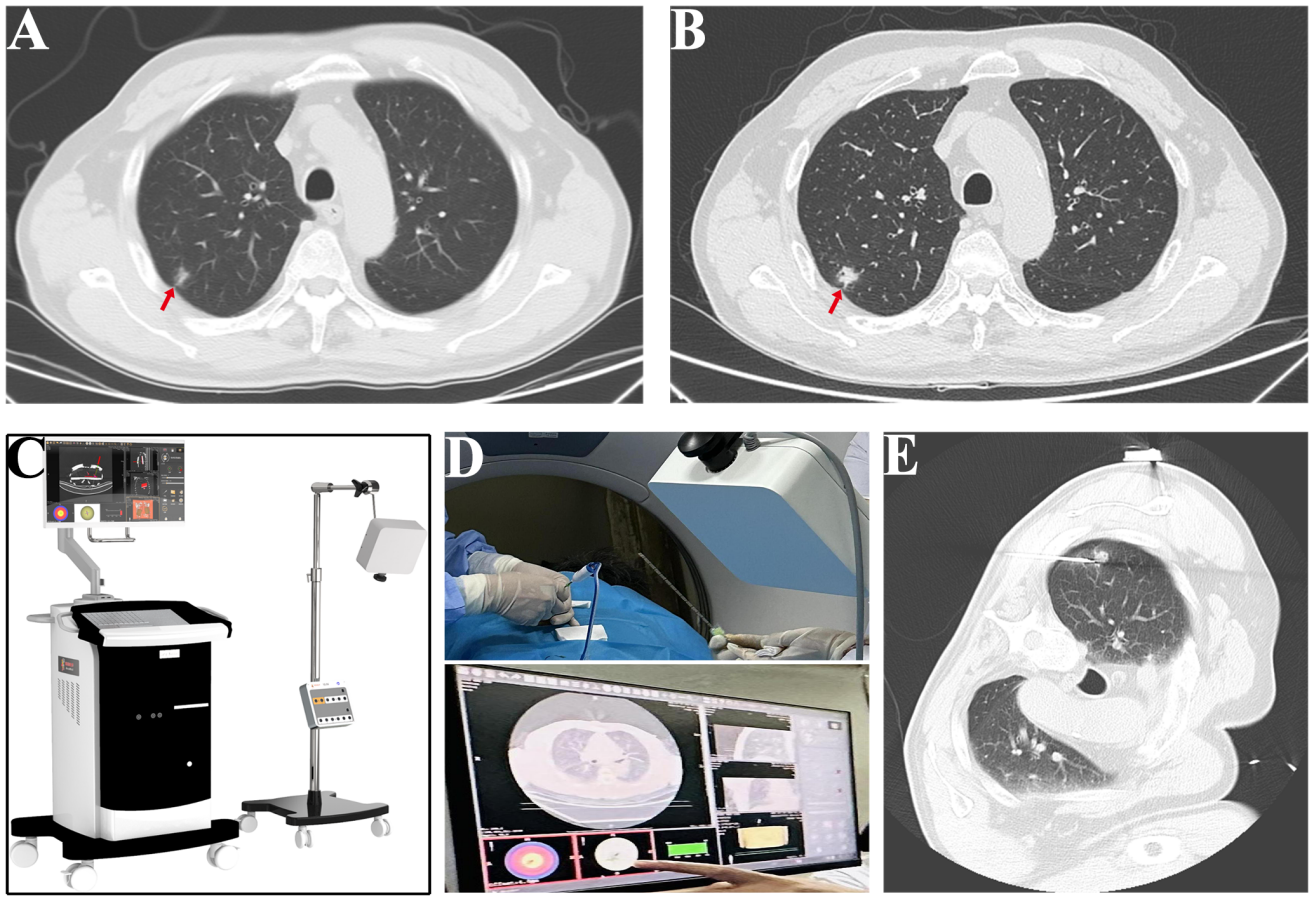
Process of CT-electromagnetic navigation system guided percutaneous laser ablation. (A) The size of the pulmonary nodule was measured at 8.5mm×6.1mm in March (red arrow). (B) The size of the pulmonary nodule was measured at 18mm×19mm in September (red arrow). (C) Image of electromagnetic navigation system equipment. (D) Representative images of the puncture process guided by CT electromagnetic navigation system. (E) Image of successful positioning of the puncture sheath to the tumor site.
